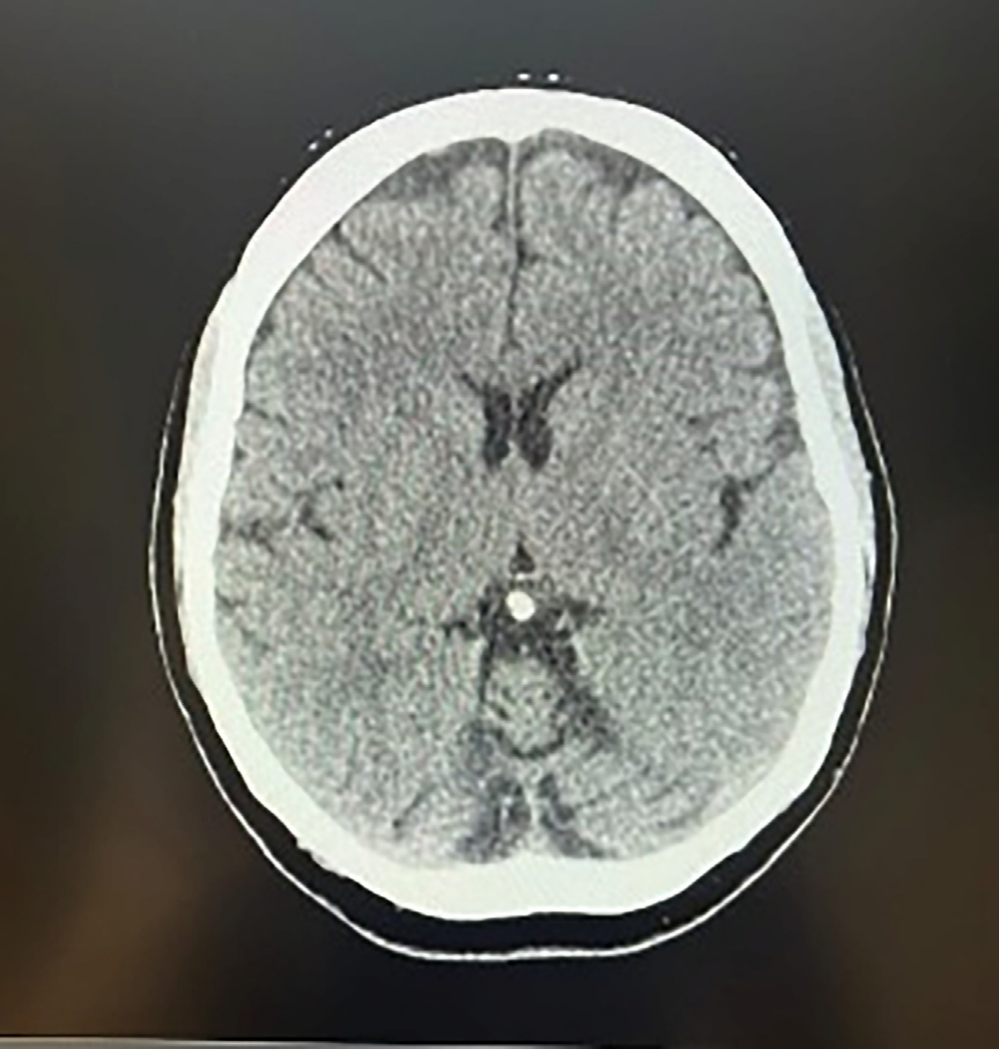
Non-contrast cranial CT scan, conducted after the onset of neurological symptoms, showed no signs of intracranial pathology.
